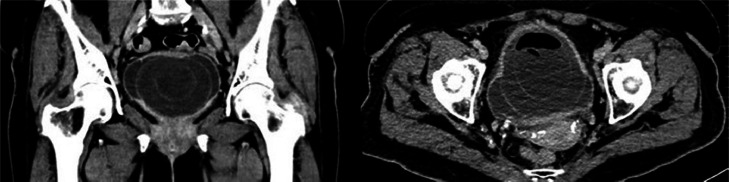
Portal phase abdominal CT: CT images displaying a bladder-occupying lesion with a liquid density core and a thin, homogeneous, nonenhancing wall, 9 cm in diameter. A notable feature is a second cystic lesion within, characterized by a similar wall and a hydroaerial level, indicative of a complex, layered structure.
